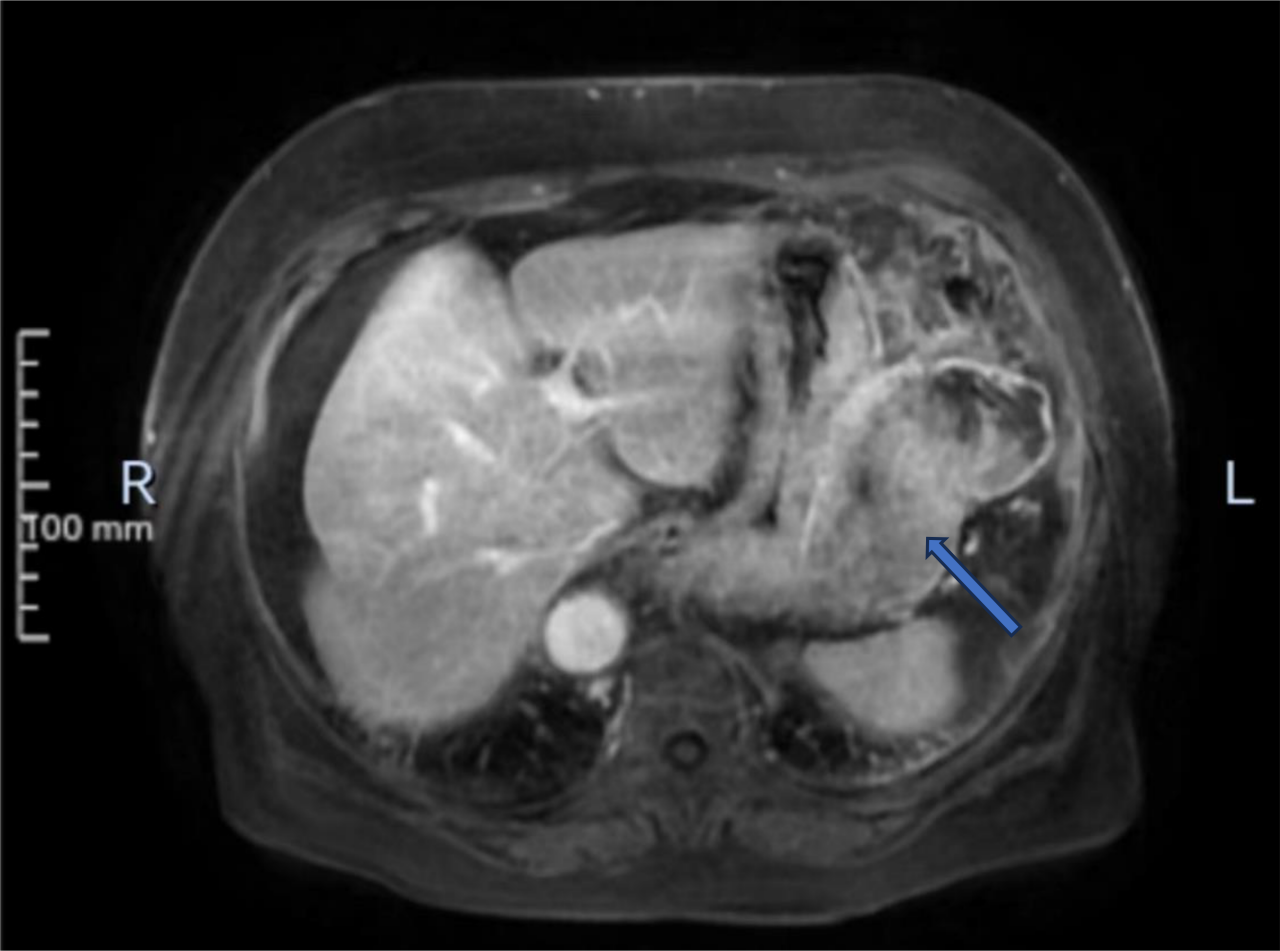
MRI of midsection, with the marked areas representing lesions.
